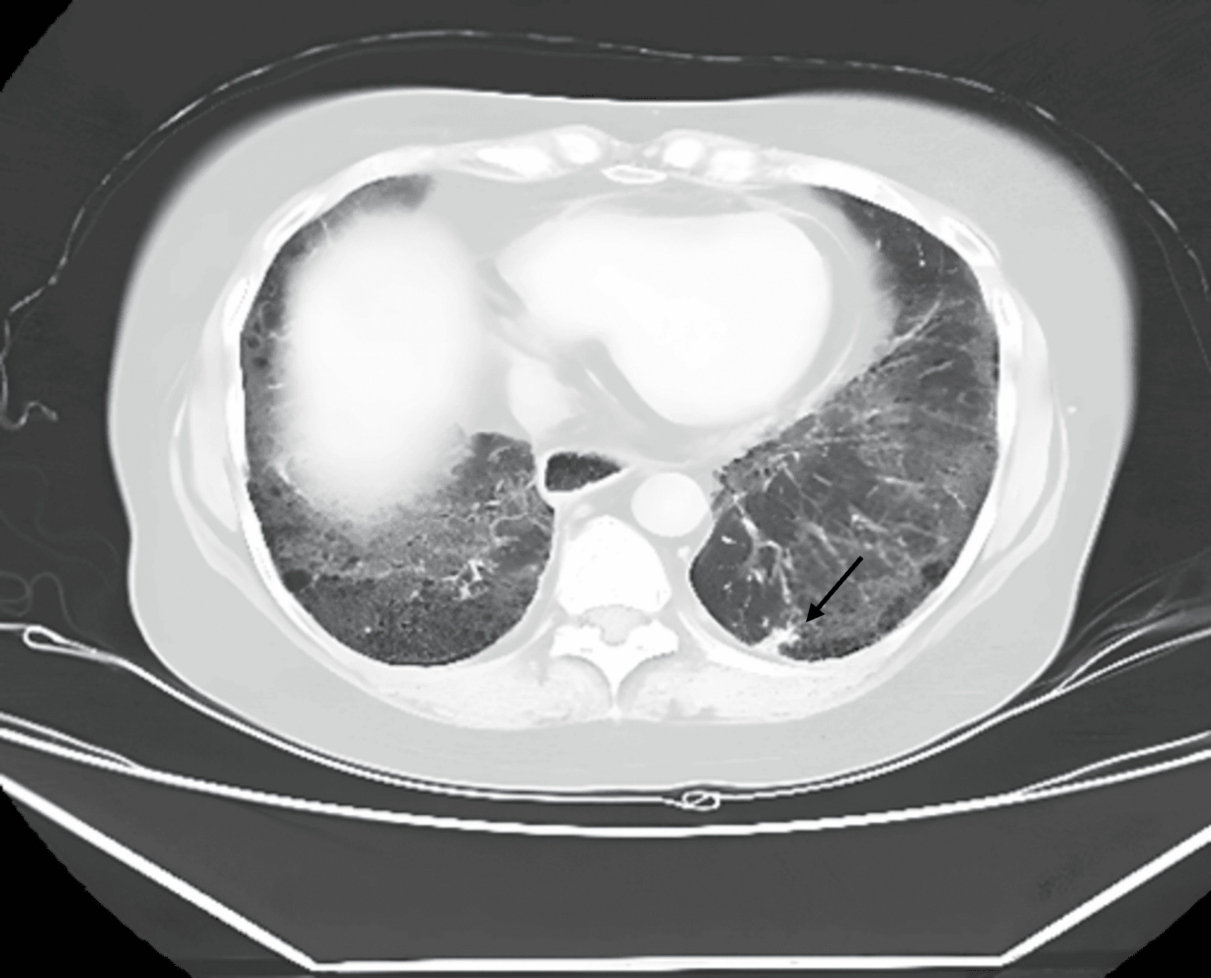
The CT of the chest shows interstitial lung disease (probable UIP)—bibasilar subpleural reticulations, interlobar septal thickening, and mild GGOs with a left lower lobe subpleural nodule (1.1 x 0.4 cm) in the posterobasal segment UIP: usual interstitial pneumonia; GGO: ground-glass opacity
